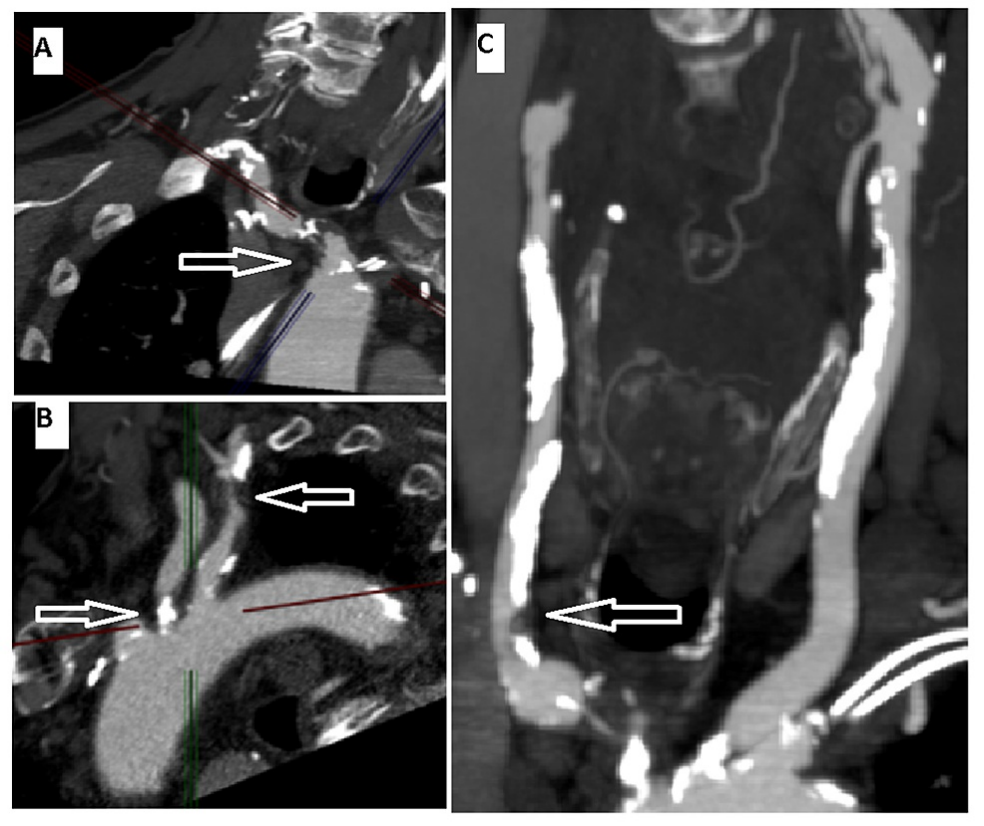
CTA of the head and neck reveals severe multivessel disease. CTA showed a severe proximal innominate artery stenosis (panel A, arrow), in addition to severe narrowing of the proximal left common carotid and subclavian arteries (panel B, arrows). In addition, there was moderate right common carotid artery stenosis (panel C, arrow). CTA, CT angiogram
